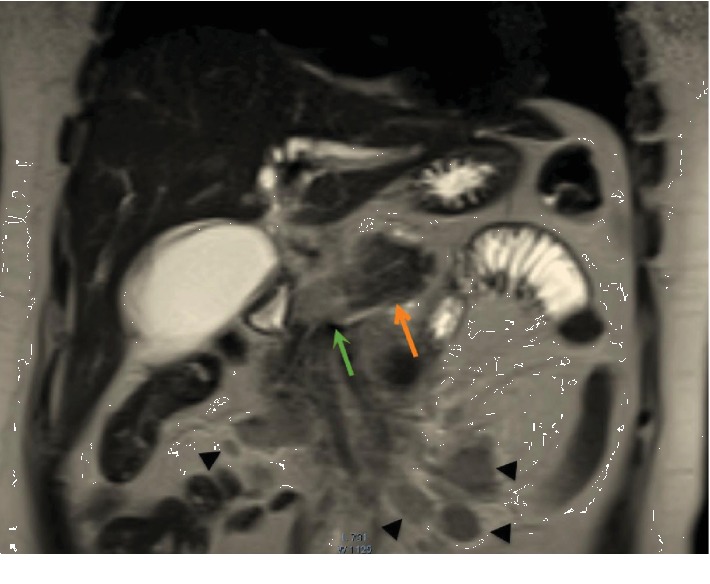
Magnetic resonance imaging (MRI) of the abdomen. Coronal slice shows the line of demarcation between the distal normal body and tail of the pancreas (orange arrow) and the neoplastic proximal body (green arrow), with multiple mesenteric and peripancreatic neoplastic lymph nodes (black arrowheads).
